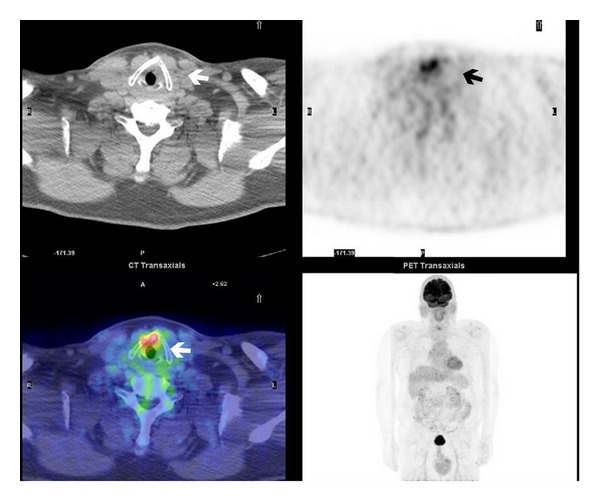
Asymmetrical ^18^F-FDG uptake appreciable in larynx at PET/CT scan (arrow).
